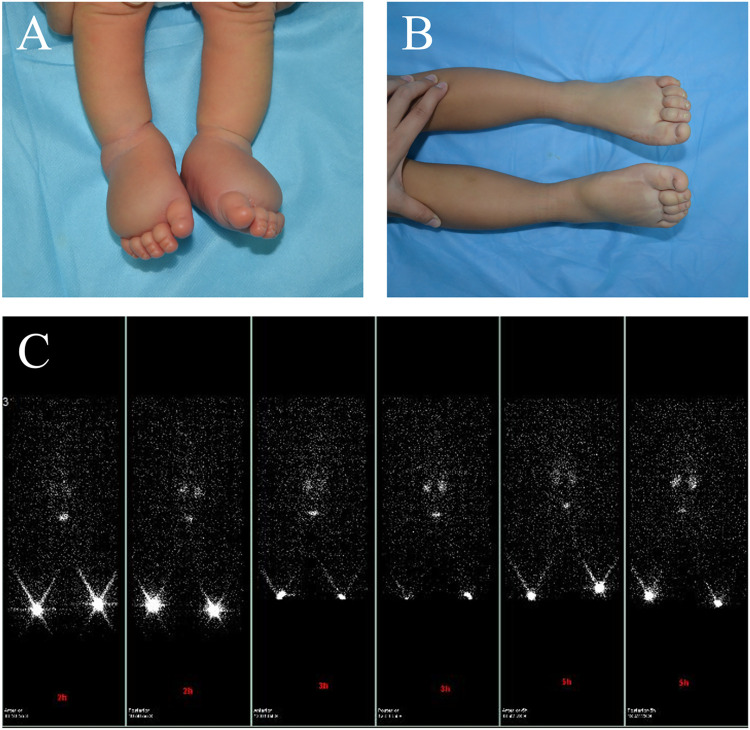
A male patient presented with primary lymphedema affecting both feet. The patient's feet were noted to be swollen during prenatal examination. (A) At birth, the patient exhibited swelling in the feet, and the Stemmer sign was positive. (B) After 3 years of physical therapy, the swelling improved. (C) Bilateral lower limb lymphoscintigraphy at age 3 revealed that the inguinal lymph nodes on both sides did not show any uptake.
